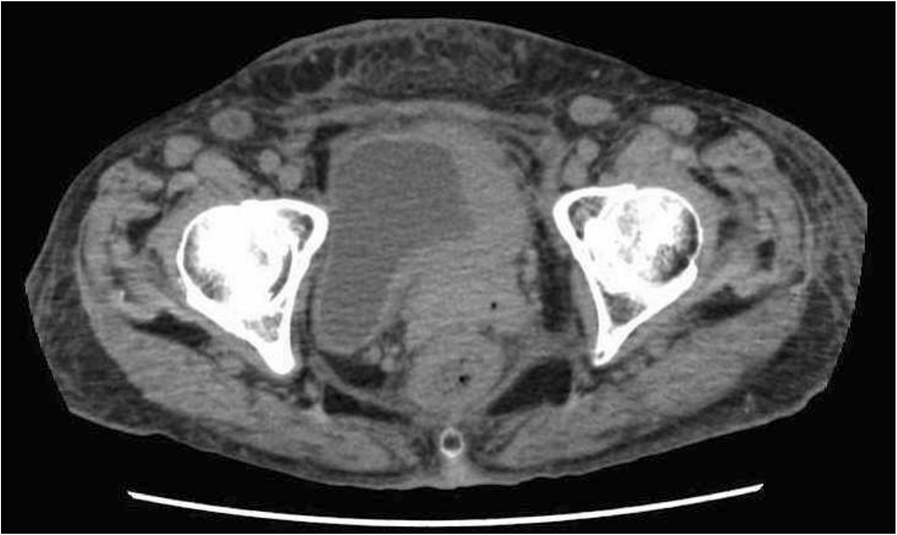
Computed tomography. Thickening of the left bladder wall
